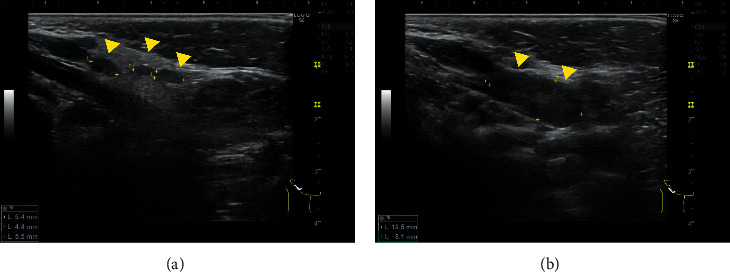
Cervical ultrasonography revealing mild reactive lymphadenopathy.
